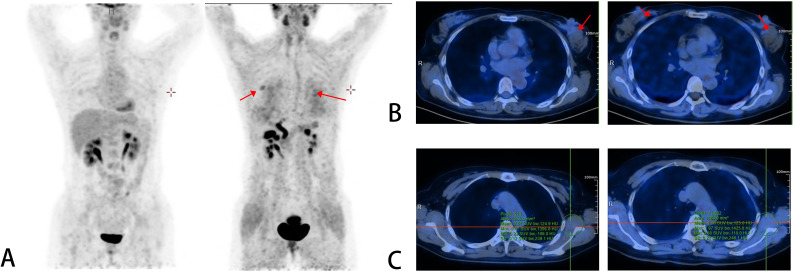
Comparison of two PET/CT images after neoadjuvant therapy. In (A–C), the left images are [^18^F]FDG PET/CT scans, and the right images are [^18^F]AlF-NOTA-FAPI-04 PET/CT scans. Additionally, residual lesions can be observed on the [^18^F]AlF-NOTA-FAPI-04 PET/CT. In both PET/CT scans, the uptake in the left axillary lymph nodes remained at background levels.
